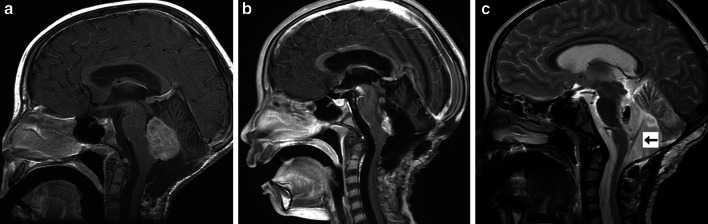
Preoperative and postoperative MRI images from patient 2. a Preoperative sagittal T1-weighted MRI with gadolinium demonstrating recurrent, enhancing tumor filling fourth ventricle. b Postoperative sagittal T1-weighted MRI with gadolinium demonstrating subtotal resection of tumor. A small amount of tumor adherent to the floor of the fourth ventricle was purposefully left behind. c Postoperative T2-weighted sagittal MRI demonstrating catheter position within the fourth ventricle. The catheter, as shown by the *arrow*, is positioned in a trajectory such that injury to the brainstem and cerebellum are avoided and all catheter holes are within the fourth ventricle
